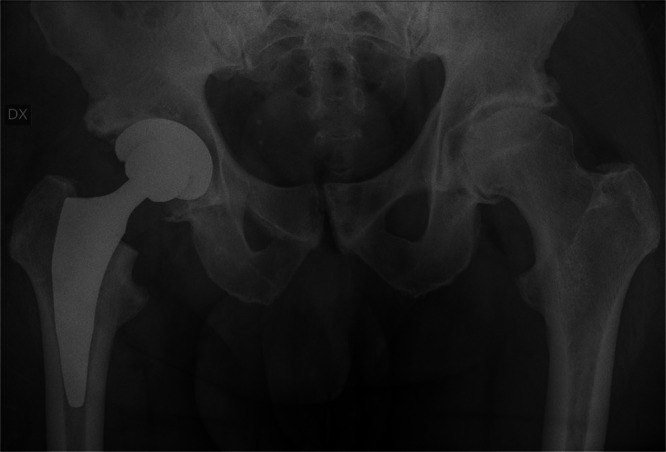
Two‐year postoperative radiographic follow‐up of a standard 3C cementless short femoral stem (CCD angle: 131°). CCD, Caput–Collum–Diaphyseal.
